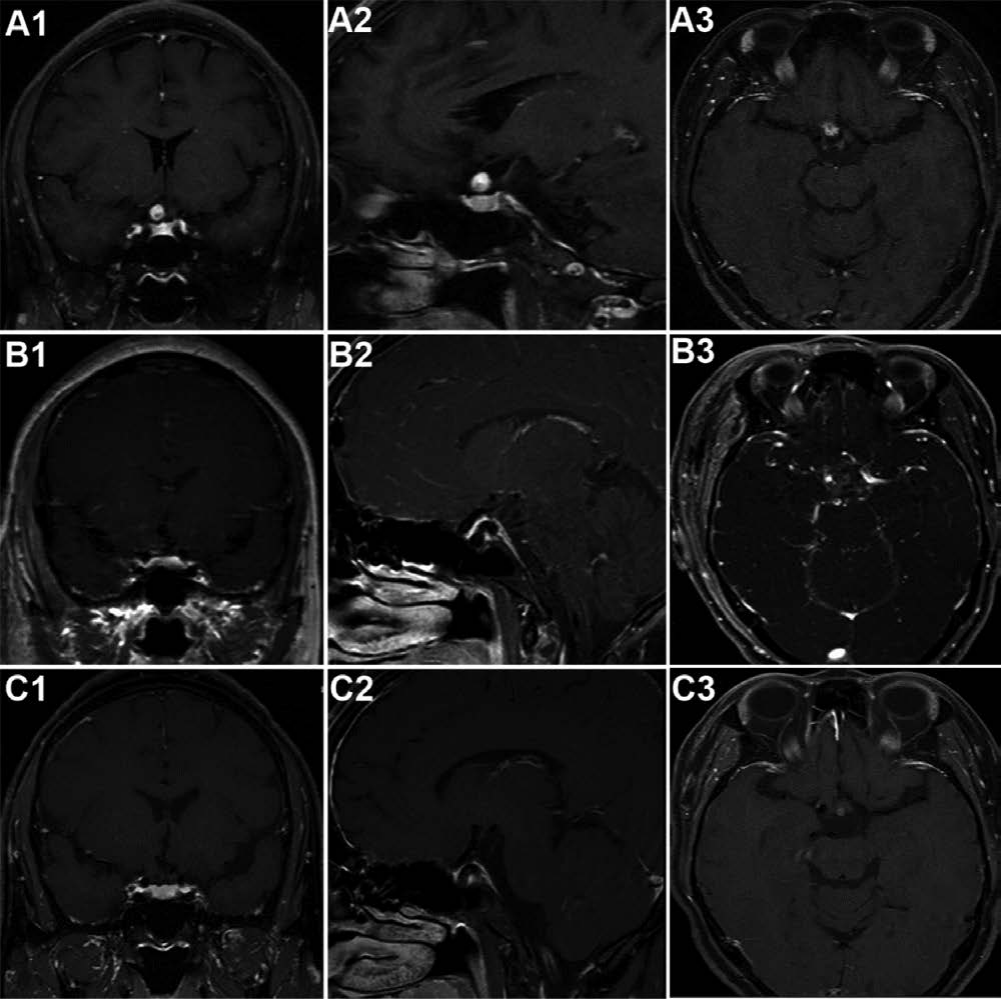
Preoperative MRI showed an enhancing nodule (1.5 × 0.9 cm) on the right side of the chiasma without orbital extension with a limited cyst behind it along the enlarged optic nerve (A1–A3); Repeat MRI affirmed no lesion residue was found 48h after the surgery (B1–B3); MRI reexamination in the 3rd month of clinical follow-up demonstrated no recurrence of the lesion (C1–C3).
